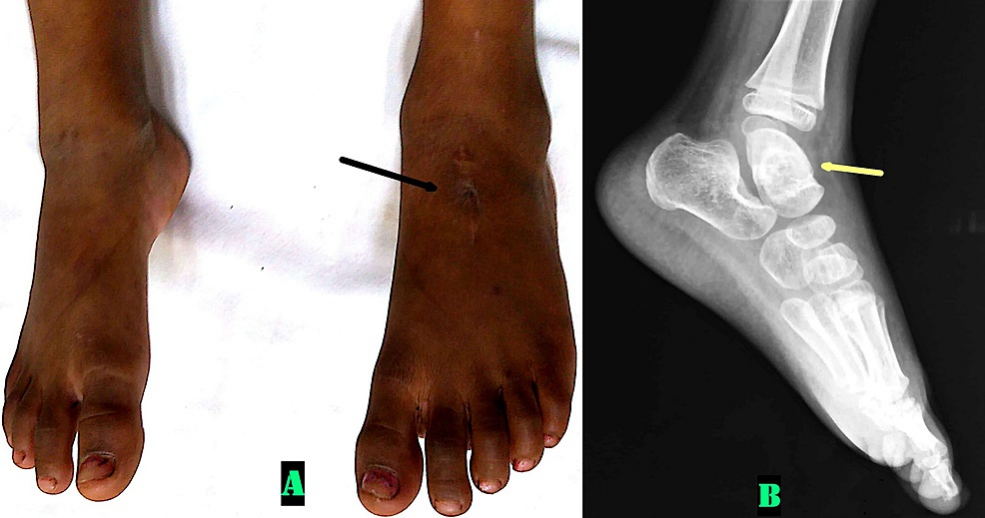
Clinical and x-ray picture at final follow-up. 4A: Clinical picture of both feet showing healed surgical scar on the dorsum of left foot (black arrow), 4B: Plain radiograph of left ankle lateral view showing complete resolution of the lesion (yellow arrow)
